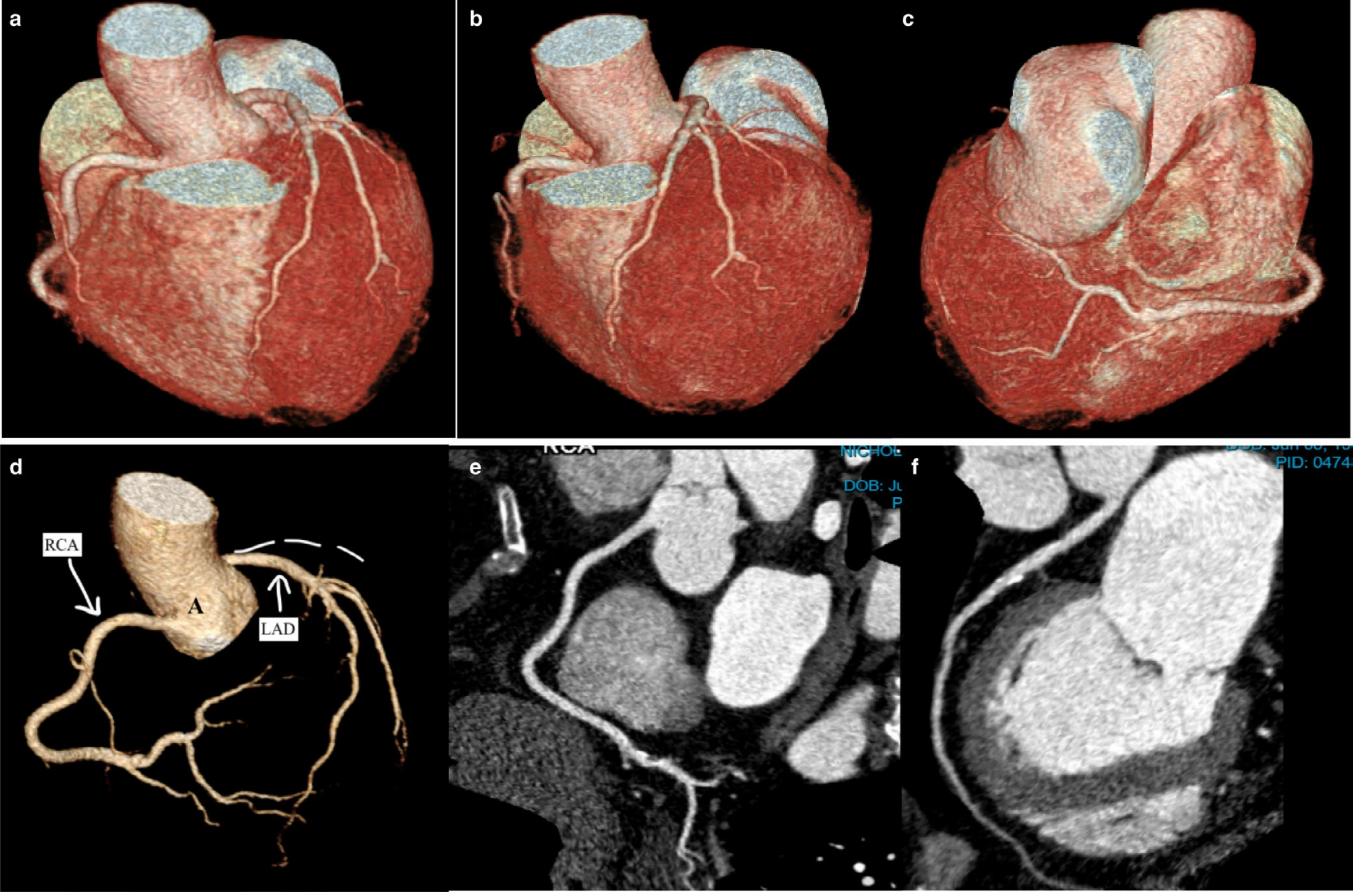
3D volume rendering coronary CT images (a–d): Panels (a–c) show the absence of the LCx, with its territory instead supplied by a hyperdominant RCA and diagonal branches of the LAD. The left main continues as the LAD, and the RCA is present with a large posterolateral branch crossing the heart's crux. Panel (d) displays the aortic root (A), LAD, hyperdominant and enlarged RCA, and absent LCx is not visible; the ordinary position of the LCx is marked as a dotted line, highlighting the single LAD and absence of LCx in the interventricular groove. Curved Planar Reformation (CPR) images Panels (e and f) depict: (e) the hyperdominant RCA crossing the crux and supplying the LCx territory, and (f) the LM coronary artery originating from the aorta and continuing as the LAD.
